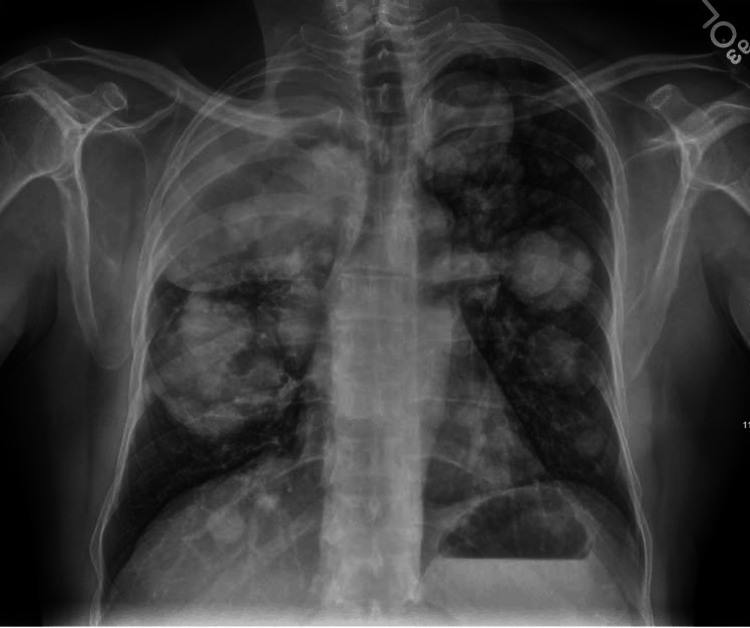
Chest radiograph demonstrating multiple bilateral pulmonary nodules in a patient with a prior solitary fibrous tumor
